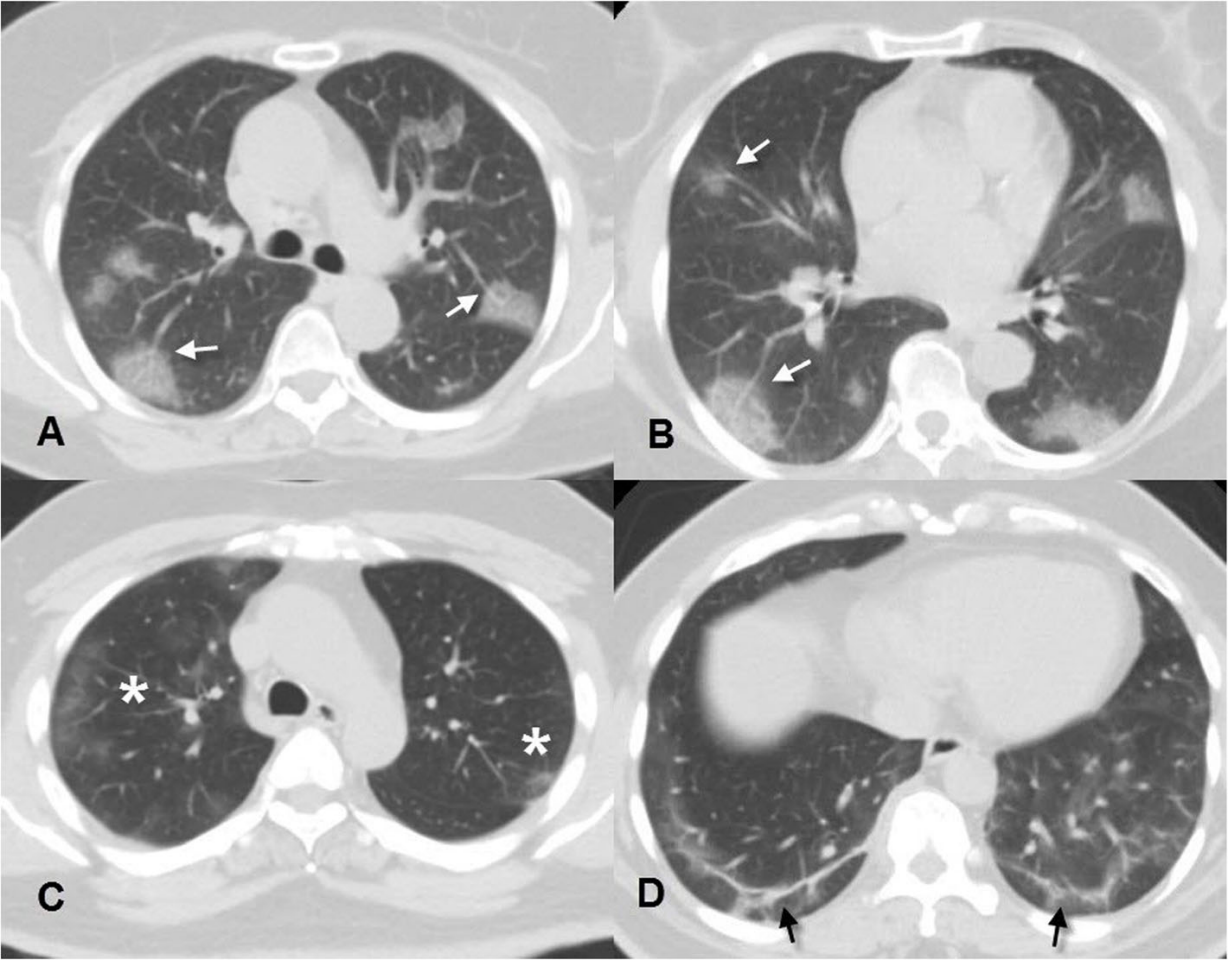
(A and B) Axial CT images, lung window, of a 60-year-old female who complained of persistent dry cough in the last 6 days. Her images displayed multiple bilateral multifocal subpleural rounded ground-glass attenuation patches with vascular dilatation (white arrows). Her initial RT-PCR was negative, then repeated because of high probability CT findings and was positive. (C and D) Axial CT images, lung window, of a 40-year-old male patient with fever and dyspnea of 10 days duration, and revealed bilateral upper lobe subpleural patchy ground-glass attenuation areas (white asterisk) as well as bilateral basal subpleural curvilinear opacities (black arrows). He tested positive for COVID-19 and received treatment at home
